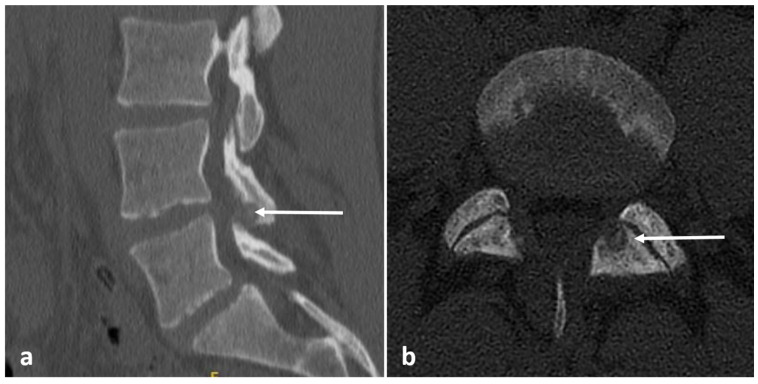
Sagittal (a) and axial (b) CT images showing an osteoid osteoma of the left L4 lamina (white arrows). The lucent nidus with central sclerotic ‘dot’ is well demonstrated. The location in the neural arch is also typical for OO.
